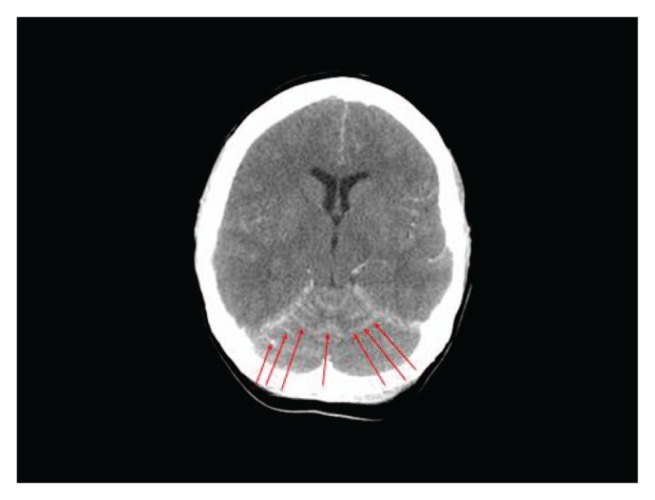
Axial postcontrast head computed tomogram demonstrating diffuse leptomeningeal enhancement involving both cerebellar hemispheres and the superior cerebellar vermis, as indicated by the red arrows.
